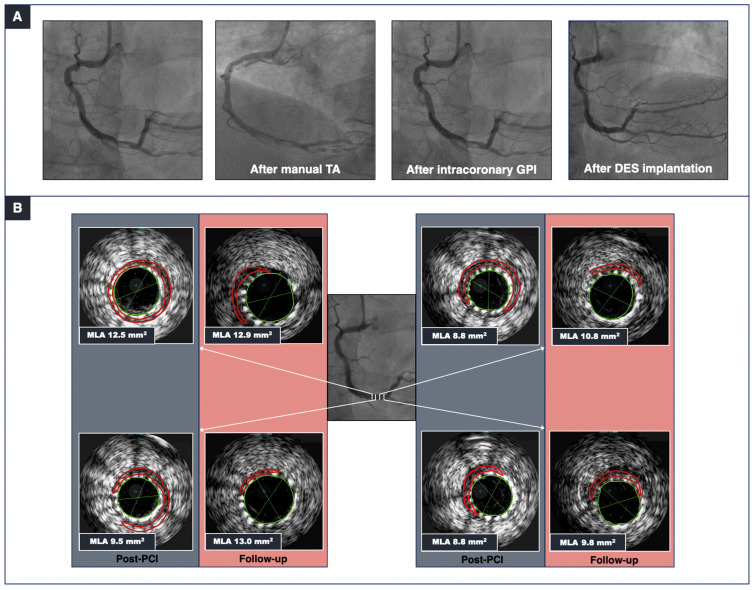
The mechanisms of stent expansion in a calcified thrombotic lesion. (A) Angiography revealed a thrombotic and calcified lesion in the right coronary artery of a patient presenting with non-ST-elevation myocardial infarction (left panel). After manual TA and intracoronary GPI administration (middle panels), a drug-eluting stent was implanted with a good angiographic result and no evidence of distal embolization. (B) IVUS (performed post-PCI and at a 5-day follow-up) documented a luminal gain 5 days after stent implantation. Abbreviations: DES = drug-eluting stent; TA = thrombus aspiration; GPI = glycoprotein IIb/IIIa inhibitor; MLA = minimal lumen area; IVUS = intravascular ultrasound; PCI = percutaneous coronary intervention.
